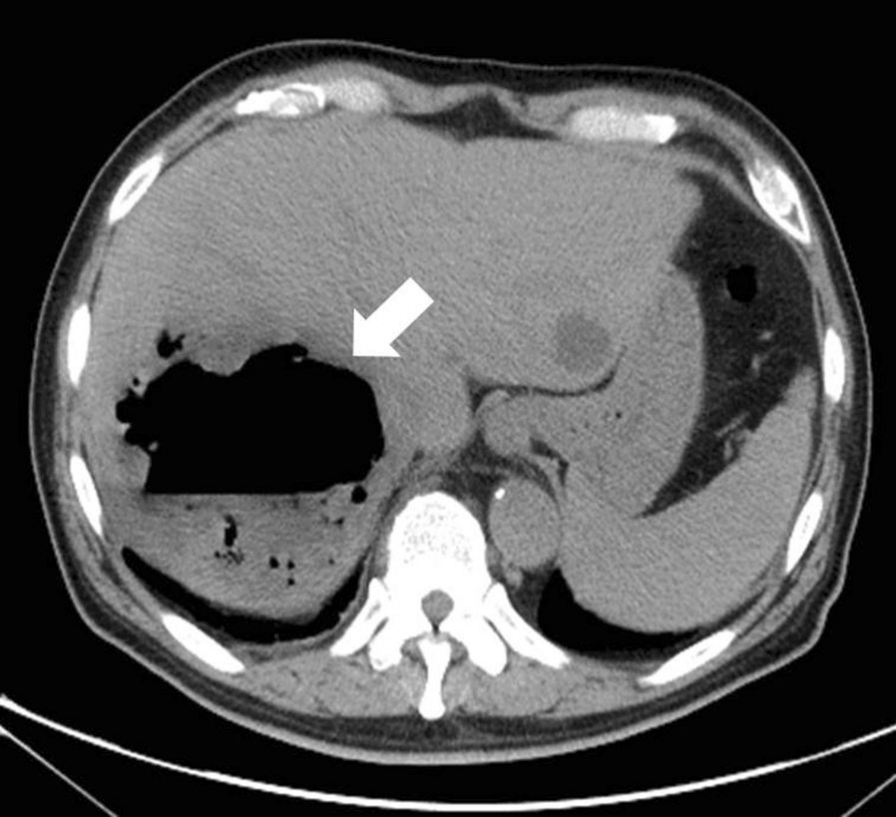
An abscess 5 cm in diameter was observed in the right hepatic lobe on abdominal computed tomography, within which gas-related fluid formation was detected (*arrow*). A cyst was observed in the left lobe
